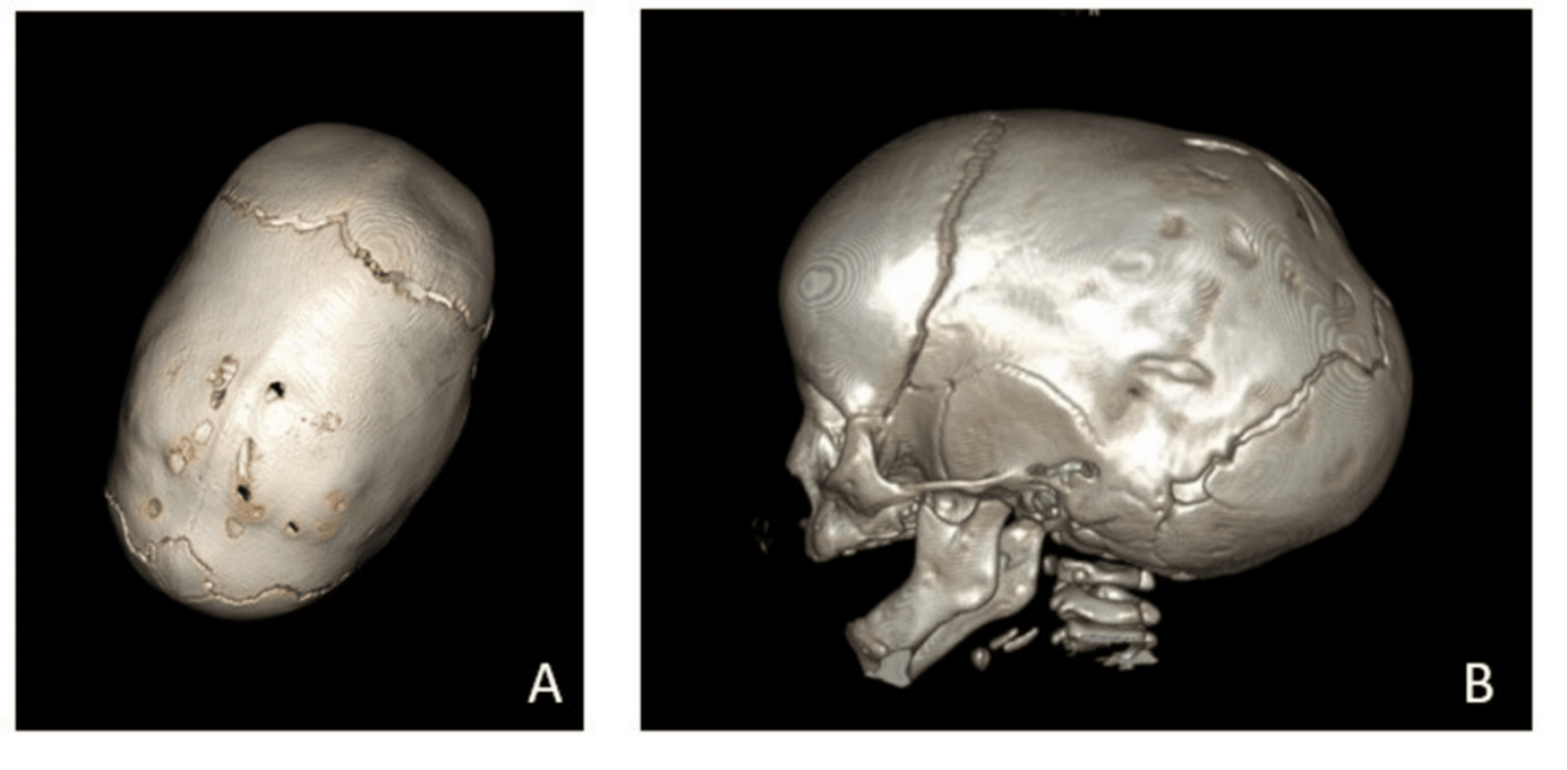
Pre-operative CT scan with reconstruction A: Vertex view; B: Lateral view
